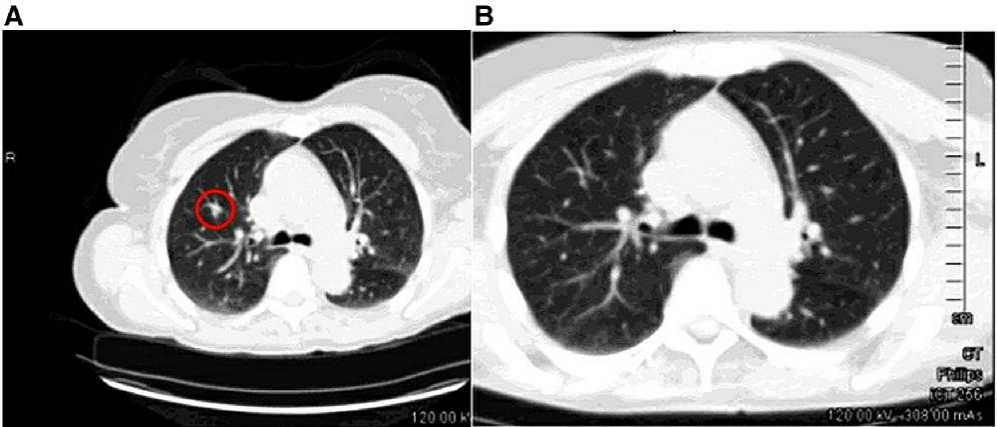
The nodule in the right upper lobe, previously detected on the CT component of the PET/CT scan (circled) (a), is no longer visible on the follow-up CT scan (B). Abbreviations: CT, computed tomography; PET, positron emission tomography.
